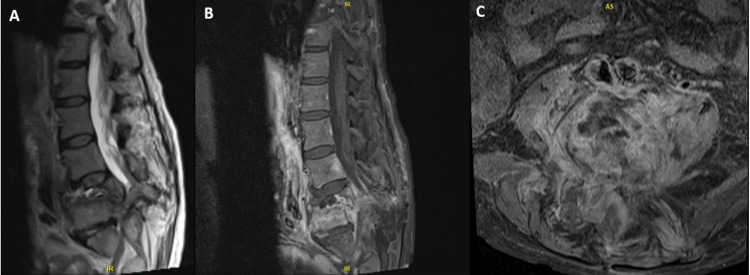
A) Sagittal T2 Turbo Spine Echo (TSE) MRI of the lumbar spine demonstrates the L4 and L5 fractures with near complete loss of the intrathecal subarachnoid space. B and C) Sagittal and Axial T1 TSE DIXON water images with fat suppression were obtained of the lumbar spine after contrast administration. Images demonstrate associated enhancement consistent with inflammatory changes but no epidural abscess or focal mass lesion.
